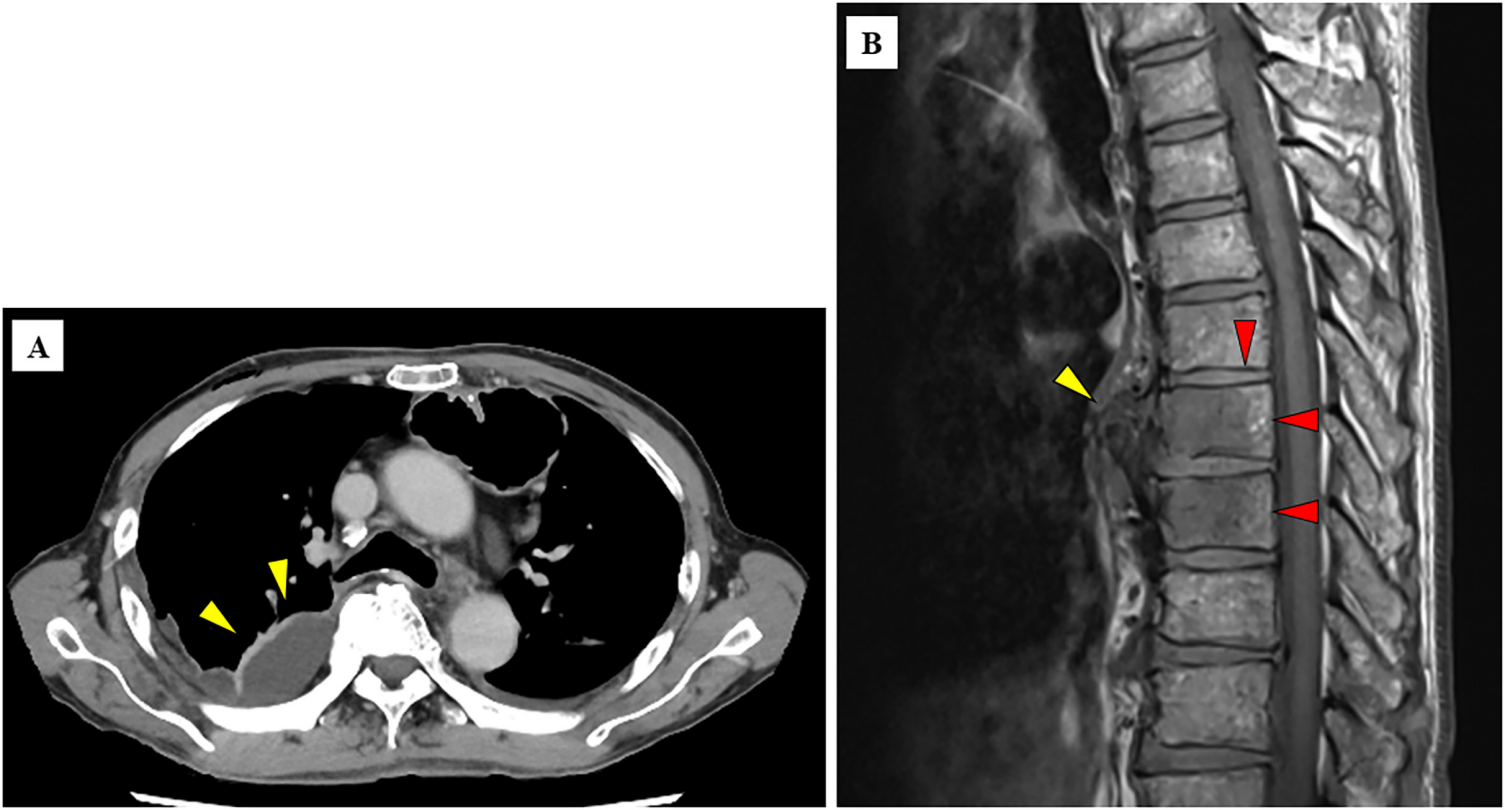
(A) Contract‐enhanced computed tomography (CT). (B) Magnetic resonance imaging (MRI). (A) Chest contract‐enhanced CT shows a right pleural effusion with a contrast‐enhancing membrane (yellow arrows). (B) MRI with T1‐weighted shows low signal localised to the anterior region of the Th 8 and 9 vertebrae body (red arrows) through osteophytes on the front of the vertebrae (yellow arrow), preserving intervertebral discs.
